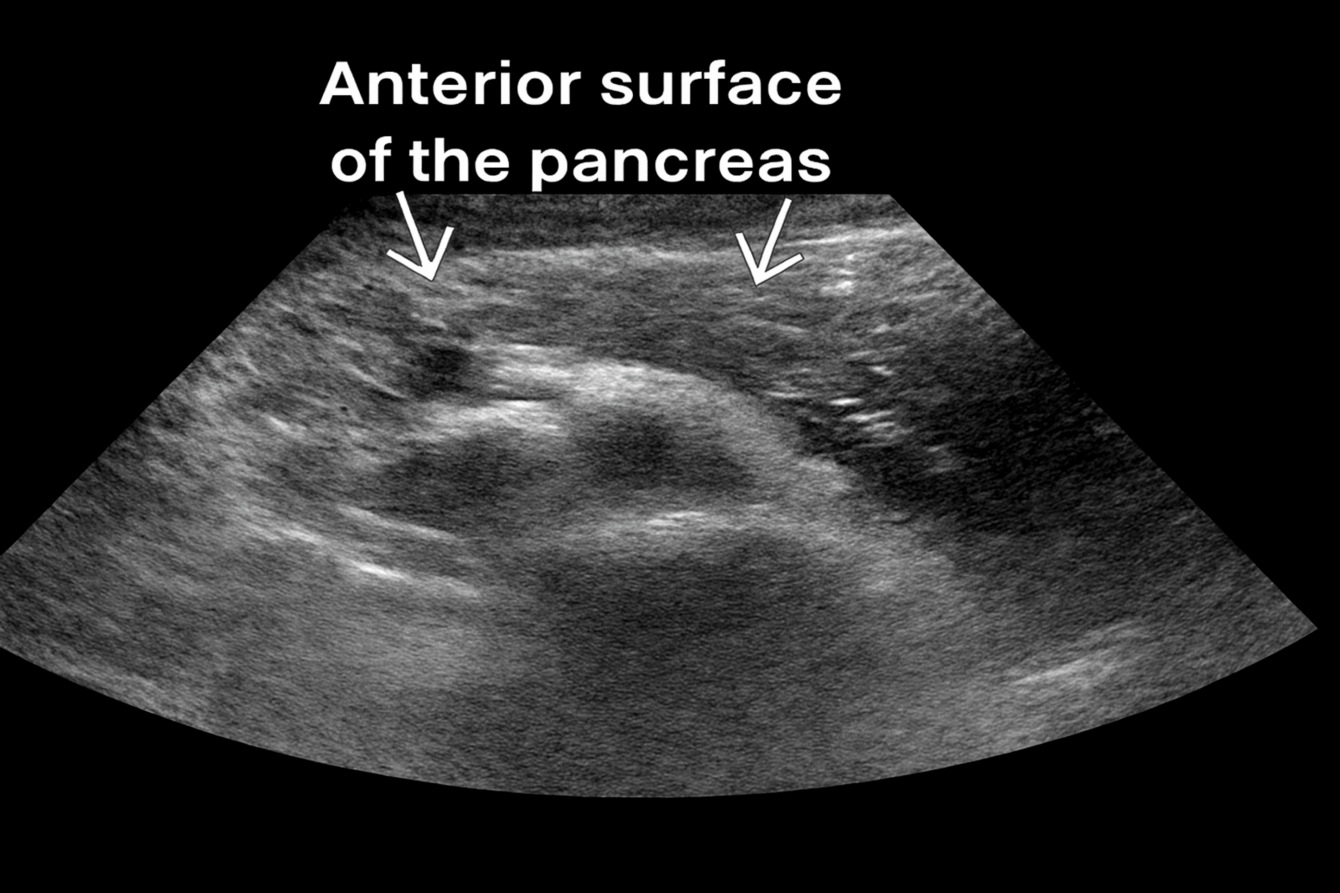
Abdominal ultrasound demonstrating pancreatic echotexture Grayscale ultrasound image displaying a heterogeneous echotexture of the anterior surface of the pancreas with increased echogenicity, suggestive of acute pancreatitis. No evidence of ductal dilatation or peripancreatic fluid collections was observed.
